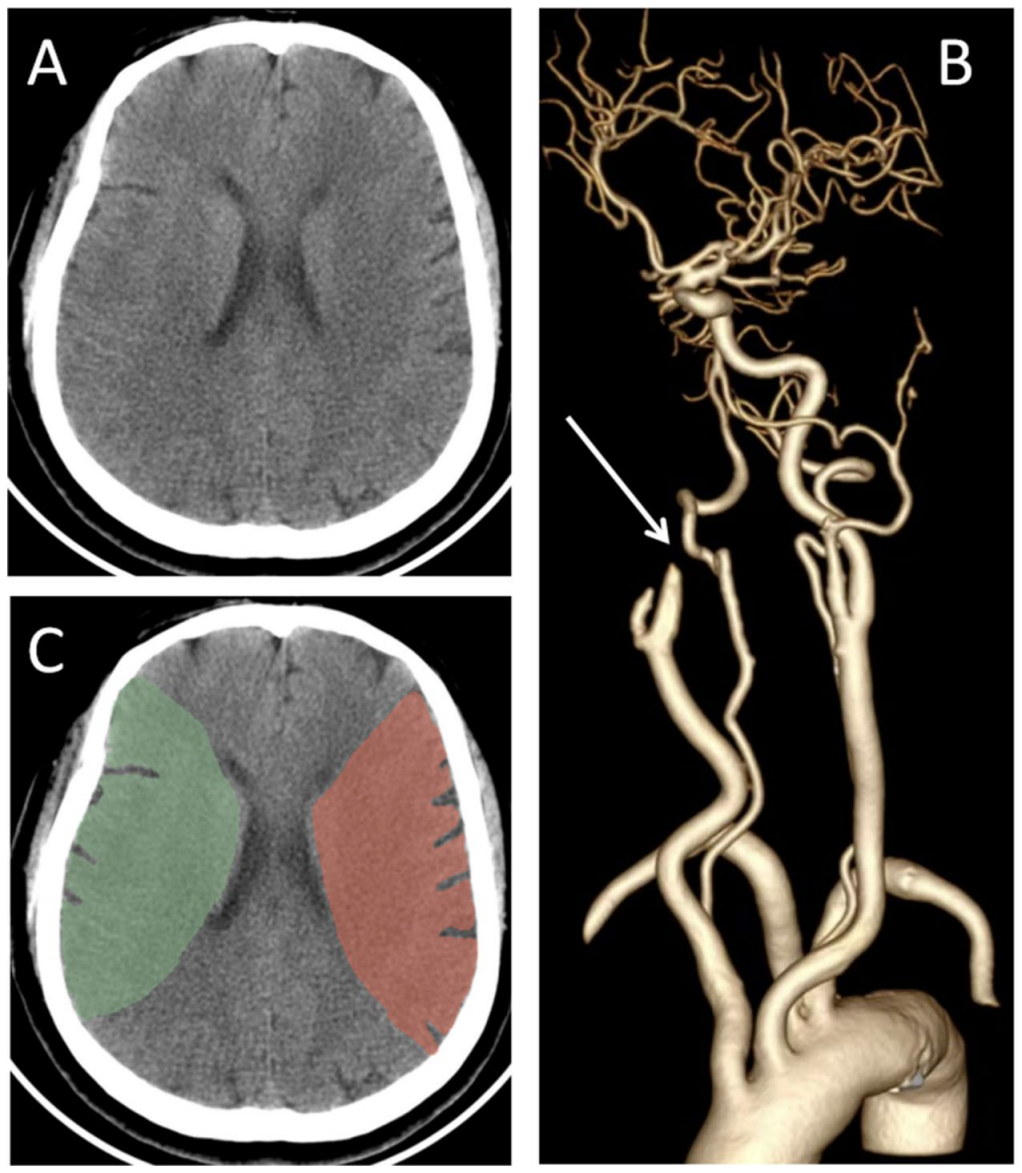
Representative cases of the NWU measurement. (A) The preoperative cranial CT of a ALVOS patient; (B) the CT angiography showed the occlusion of the right internal carotid artery; (C) the NWU was calculated by (1- density of affected territory[green region]/density of contralateral territory[red region]) × 100%. ALVOS indicates acute large vessel occlusion stroke; NWU, net water uptake.
